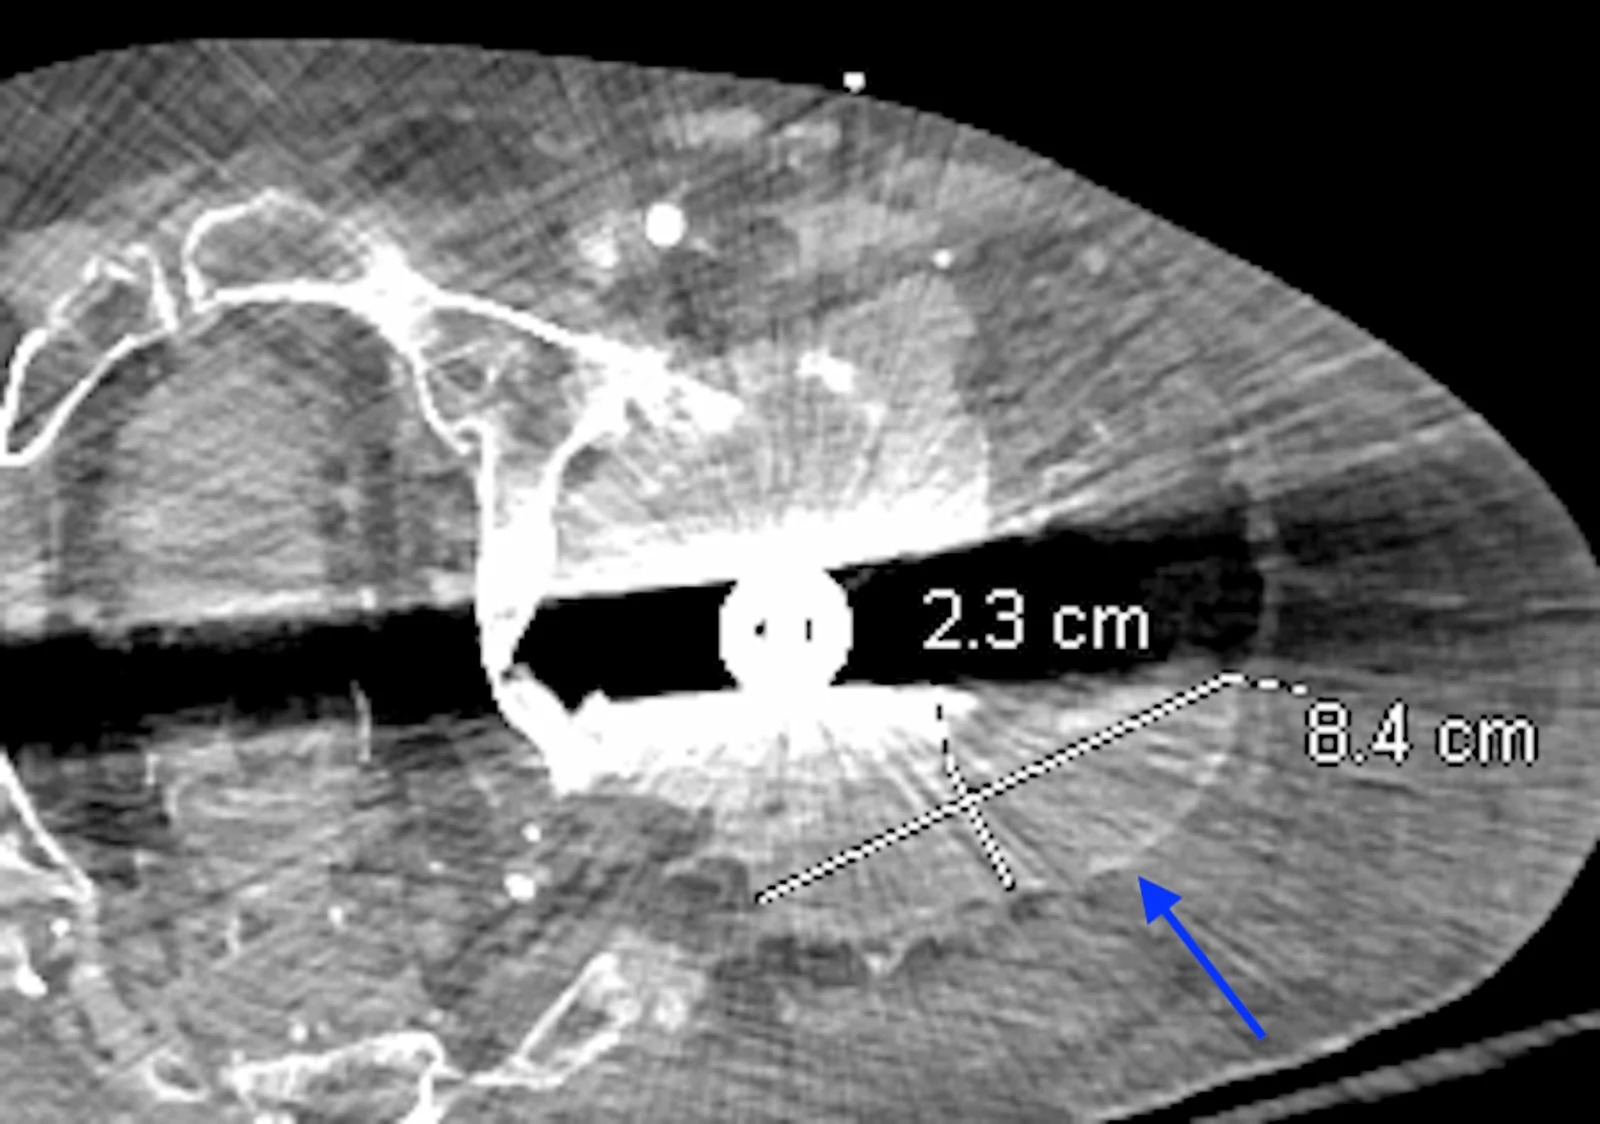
Axial CT angiography on soft tissue window. The image reveals an additional, separate fluid collection with an enhancing rim (blue arrow) posterior to the left acetabulum.
